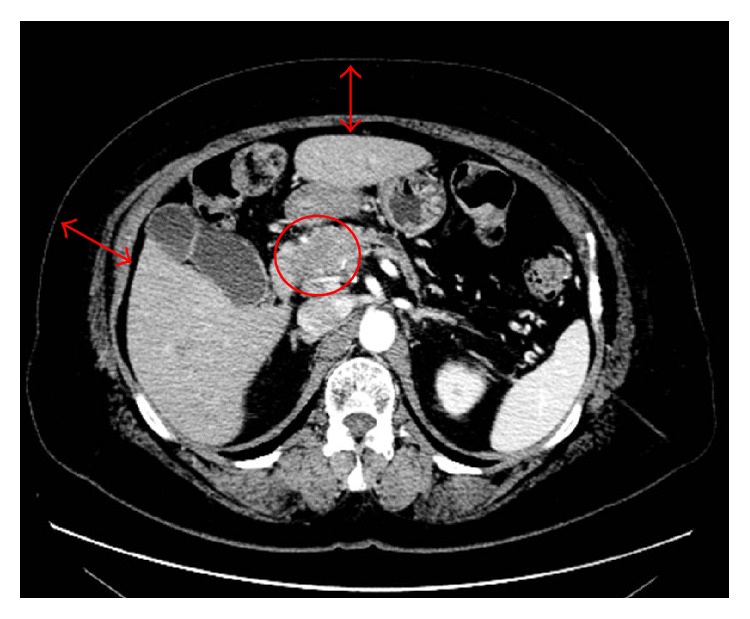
A hypoenhanced, irregular-shaped mass in the pancreatic head (*red circle*), as visualized by CT. The distance from the skin to the liver surface exceeded 4 cm (*red arrows*).
